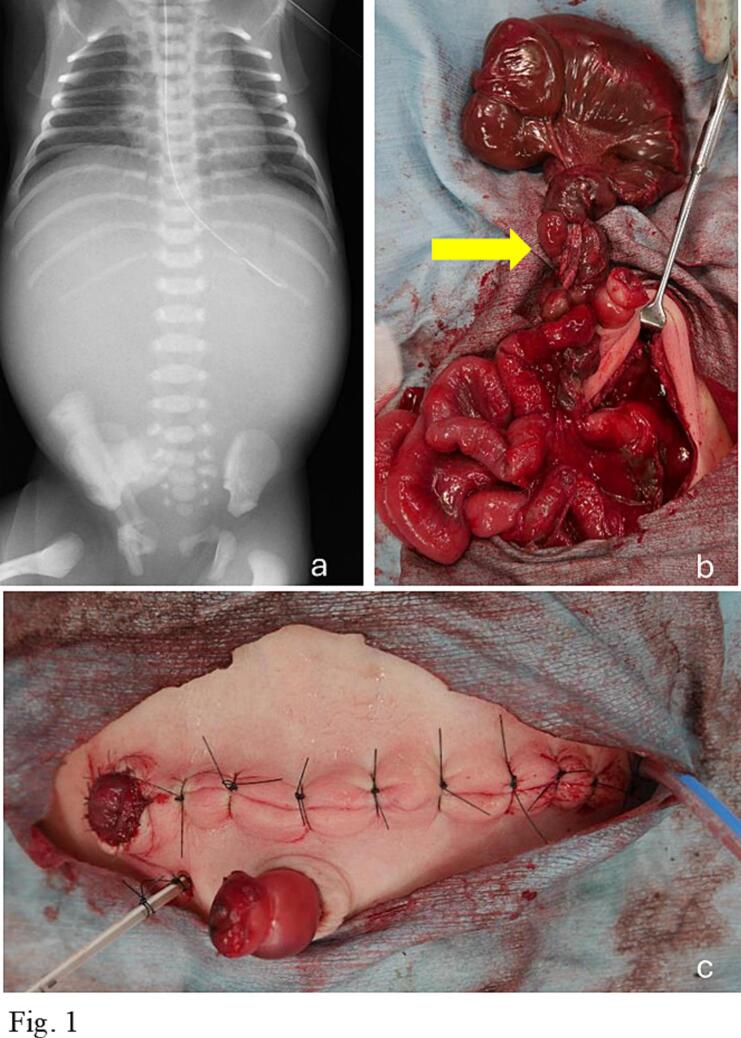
a: Abdominal X-ray without intestinal gas or free air. b: Intraoperative findings: A small bowel volvulus was found 2 cm distal to the ileocecal valve with a 1080-degree rotation. The yellow arrow (↓) shows the pedicle of the intestinal volvulus. c: Postoperative findings: A proximal end ileostomy and a distal tube ileostomy were created. A 6 Fr feeding tube was placed in the ascending colon. (For interpretation of the references to colour in this figure legend, the reader is referred to the web version of this article.)
